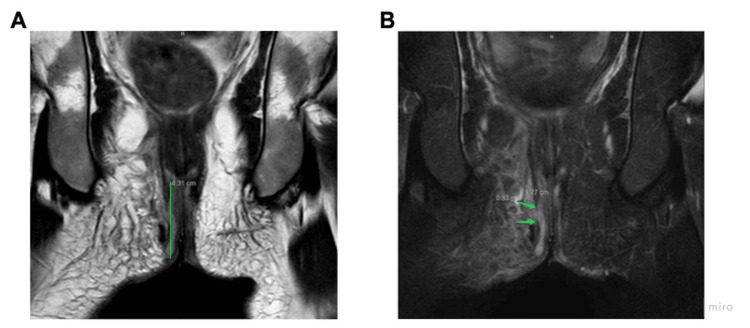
Pelvic MRI revealing (A) a perianal fistula (green line) measuring 4.31 cm, and (B) a rim enhancing collection measuring 1.3 cm x 0.33 cm (green arrows) with surrounding packing material.
